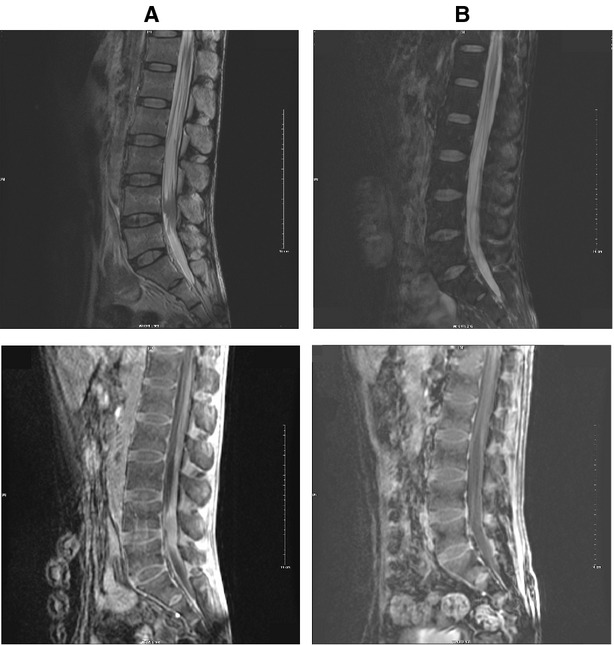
In Patient 3, MR scan shows a mass lesion on the lumbar spinal canal (A). After two cycles of chemotherapy, MR images show the lesion disappeared (B).
